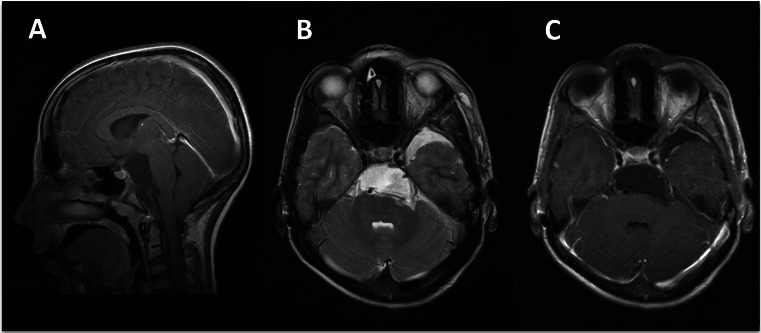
Postoperative MR images. A complete tumour removal is shown on the a parasagittal T1-weighted sequence, b axial T2-weighted sequence and c axial T1-weighted sequence after administration of gadolinium contrast
